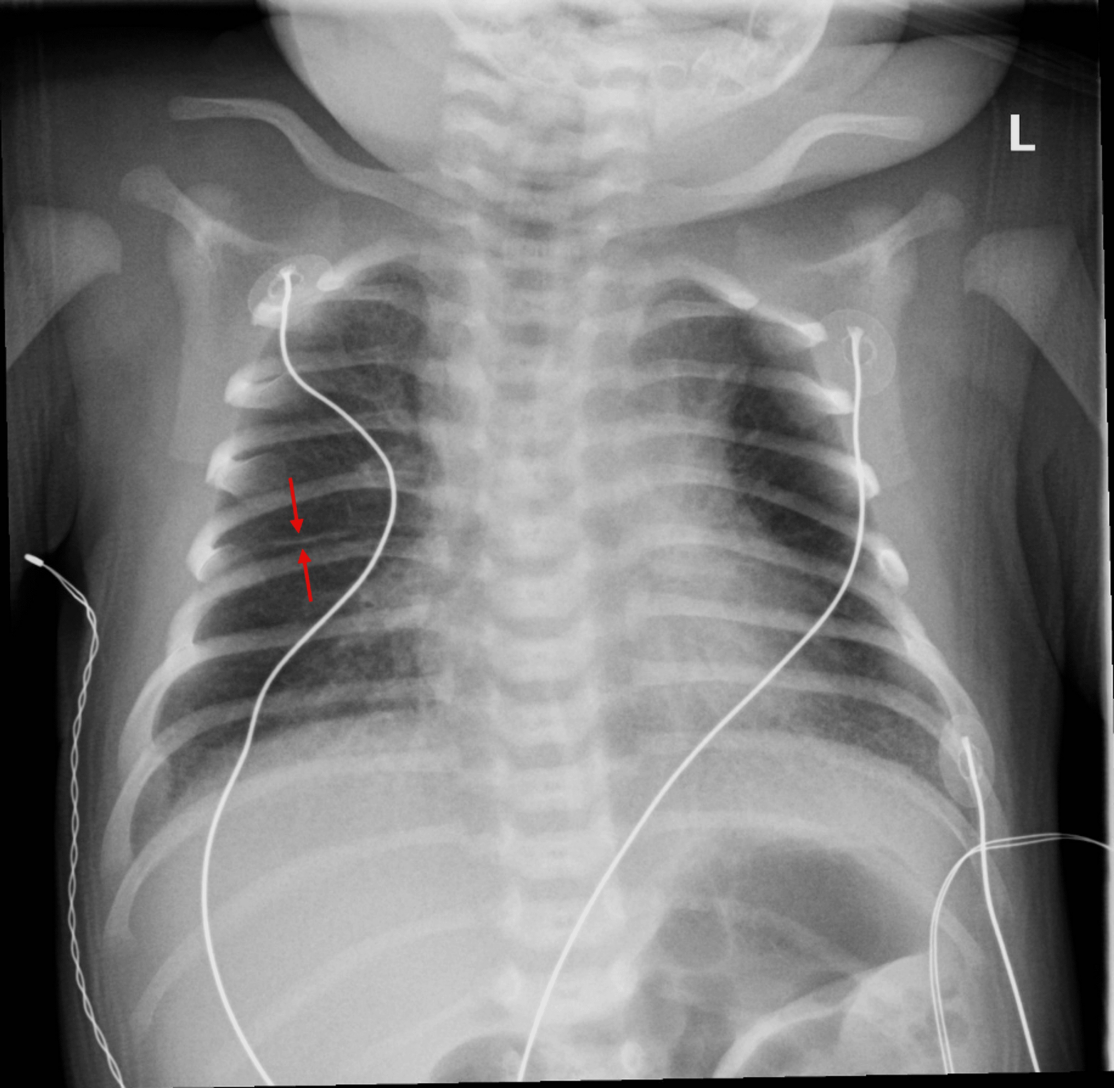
Neonatal chest radiograph Chest radiograph was ordered after continued neonatal respiratory distress and revealed bilateral perihilar streaking extending out into the lung fields with fluid in the minor fissure (red arrows)
